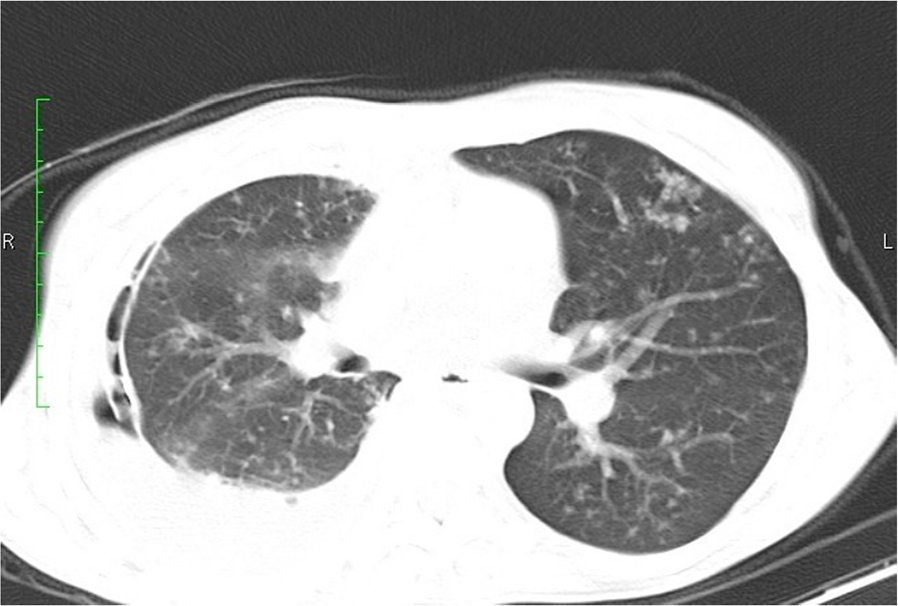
CT Chest; pulmonary window. Post chest tube insertion for right pleural effusion. Multiple subcentimeter lung nodules are seen with a ‘tree in bud’ appearance representing active endobronchial spread of disease.
